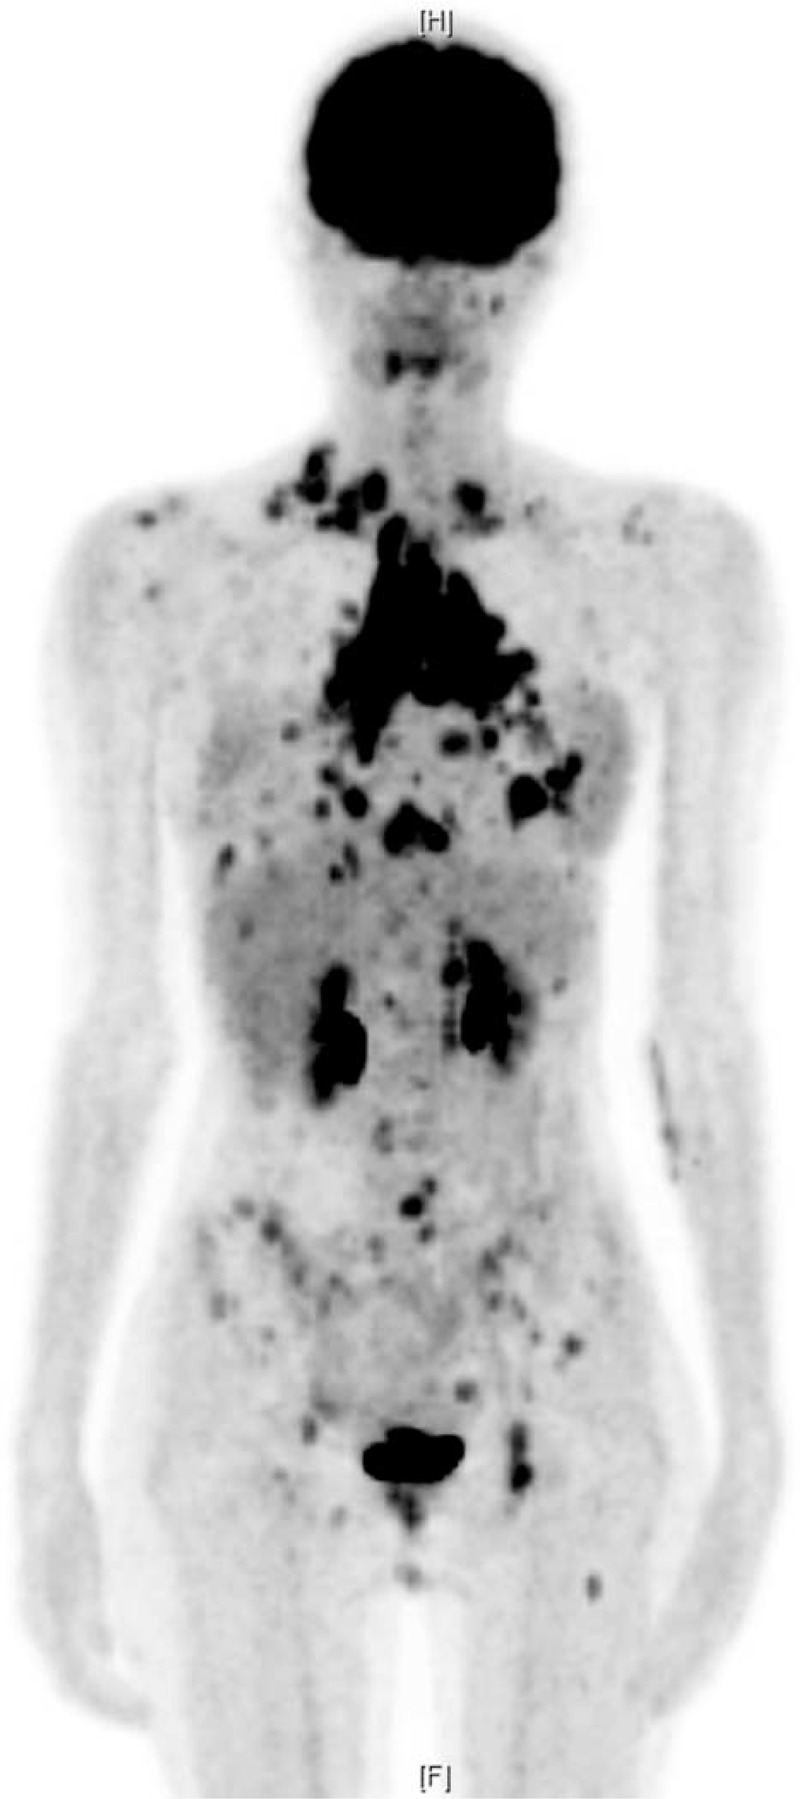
A positron emission tomography-computed tomography scan on July 18, 2014, revealed extensive [^18^F]fluorodeoxyglucose uptake within lymph nodes in the bilateral supraclavicular, mediastinal, pulmonary hilar, retrocardiac, cardiophrenic, gastrohepatic, abdominal paraaortic, left lower pelvic regions; bones in the left mandible, skull, spine, pelvic bones, bilateral scapulae, ribs, left femur; bilateral lungs and pleura; and pancreatic tail.
